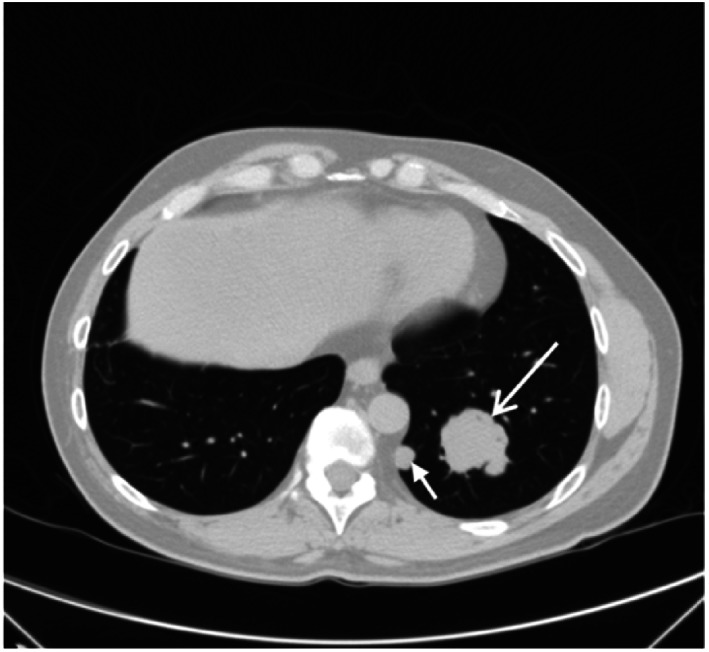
Axial CT scan of the chest in lung window showing a lobulated left lower lobe mass (long arrow) and a smaller adjacent pleural nodule (short arrow) indicative of metastases.
